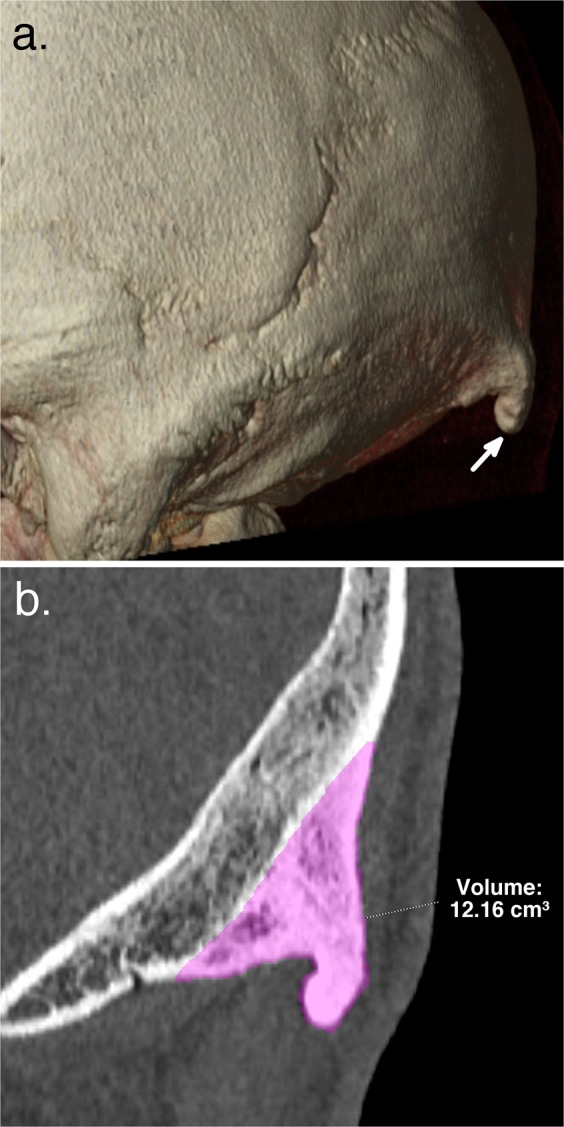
EOP measurement method. Panel a. shows volume rendering (VR) of the skull CT with a type 3 EOP (arrow) in a male patient from the 2019 dataset; Panel b. shows the corresponding measurement technique of the volume of the EOP, using the “Freehand V.O.I.” tool (SyngoVia). This EOP enlargement was the largest among all examinations, with a volume of 12.16 cm^3^.
